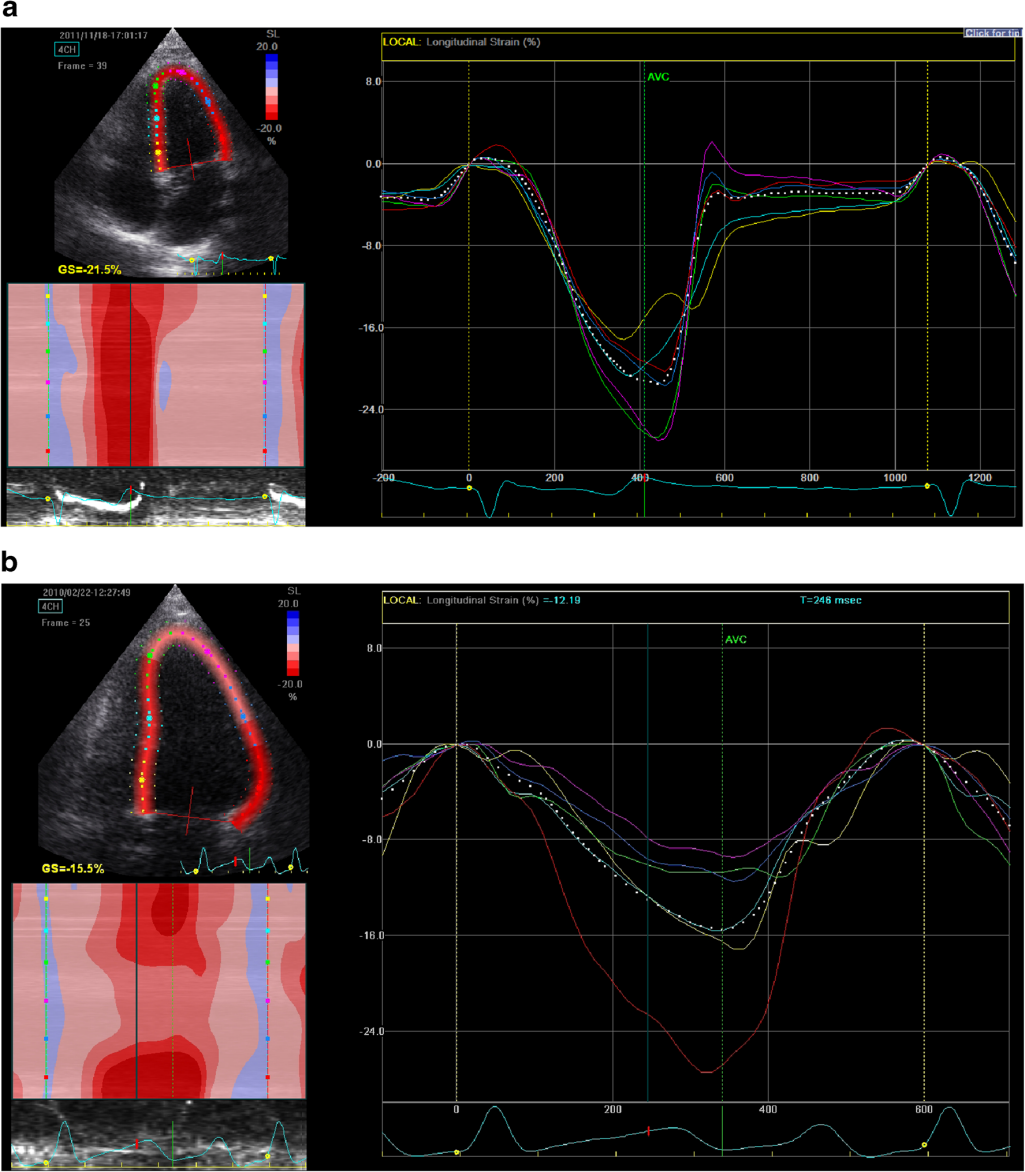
Two-dimensional speckle-tracking echocardiography (STE) for left ventricular (LV) longitudinal strain (LS) in a healthy control subject (a) and a child with end-stage renal disease (ESRD) (b). The mean LS (*white dotted line*) is the mean of the six segments of the myocardium of the left ventricle, (e.g.* yellow* basal septum,* light blue* mid septum,* green * apical septum,* red * basal lateral,* dark blue * mid lateral,* purple* apical lateral). The mean LS can be seen to be significantly lower in the child with ESRD (b) than in the healthy control subject (a)
